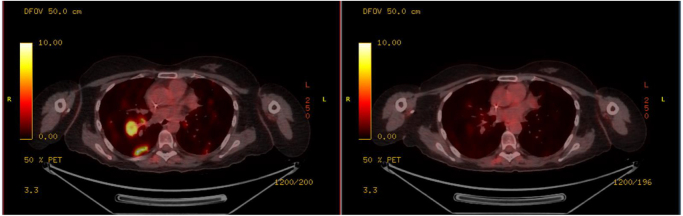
Interval axial FDG-PET/CT following treatment with trastuzumab deruxtecan showing marked reduction in FDG uptake, indicative of a partial metabolic response.
